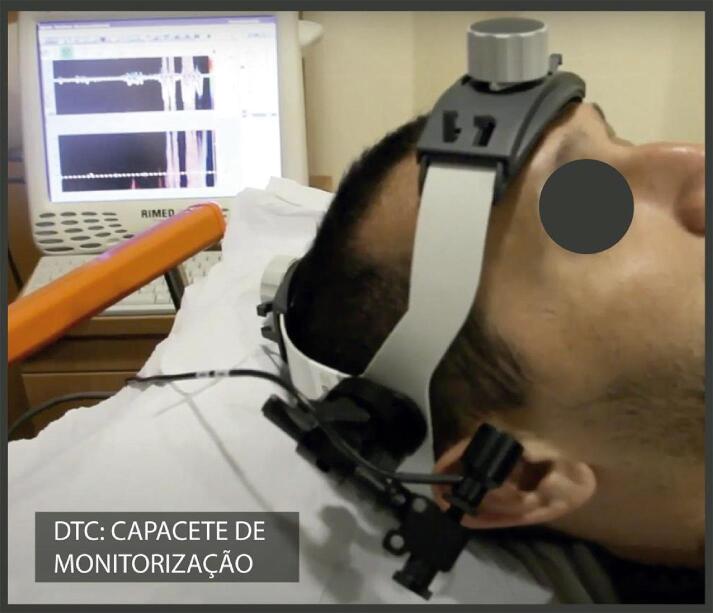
Capacete ajustável ao crânio para a fixação de transdutores e monitorização contínua de fluxo em cerebrais médias. DTC: Doppler transcraniano.
